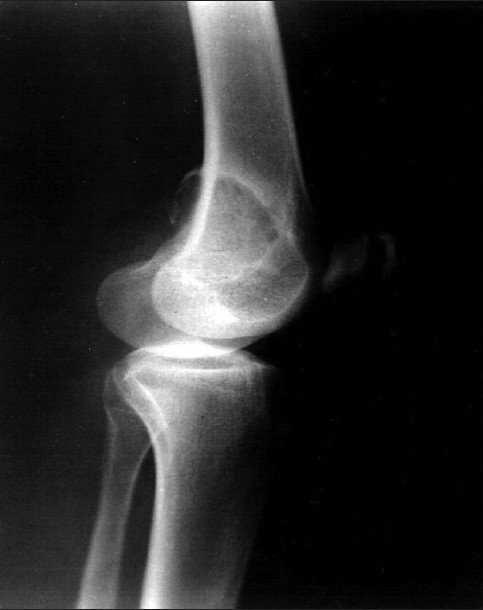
Radiograph showing, well-defined lytic, eccentric, expansile lesion with sclerotic rim in the epimetaphyseal region at the lower end of femur
